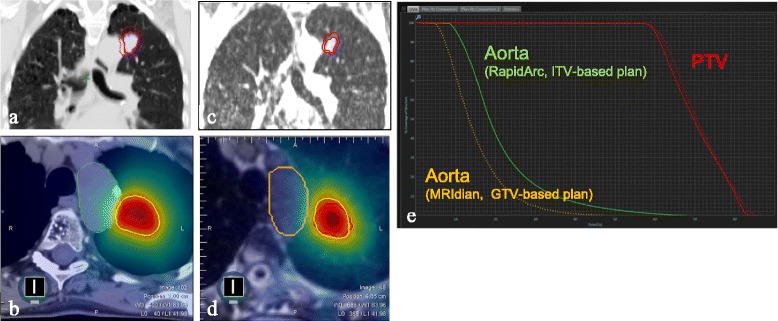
Comparative treatment plans for MRI-guided radiotherapy using breath-hold versus a standard free-breathing internal target volume (ITV)-based approach for a central tumor in a patient with interstitial lung disease. Panel a shows the ITV (7.8 cc) for a RapidArc (volumetric modulated arc therapy) plan, to which a 5 mm margin was added to derive a planning target volume (PTV, 26 cc); panel b the corresponding dose color-wash for an 8 fraction stereotactic ablative radiotherapy scheme to 60 Gy. Treatment was delivered using on-line MRI guided breath-hold on the MRIdian in which the target was the gross tumor volume (6.9 cc, Panel c), to which a 3 mm setup PTV margin was added (PTV 13.6 cc). Panel d shows the MRIdian dose color-wash, and Panel e the dose volume histograms for the adjacent aorta for both plans
